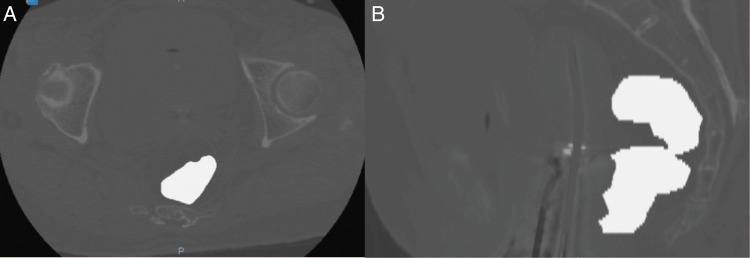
Typical CT images after replacement of internal and external CT values for the rectum and tandem/ovoid applicator. To eliminate the effects of metal artefacts on the CT images, the CT values within a rectal contour or tandem/ovoid applicator were replaced by 400 or 0 HU, respectively, and the CT values outside the rectal contours were multiplied by 0.01. Abbreviations: CT = computed tomography.
